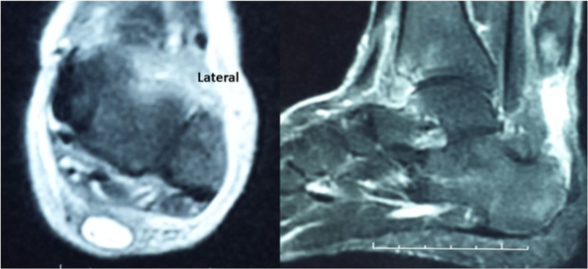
Preoperative MR images axial T2 fat sat. (*left*) and sagittal T2 fat sat. (*right*) show a complete tear of the Achilles tendon with a gap about 2.4 cm filled with fluid signal intensity
